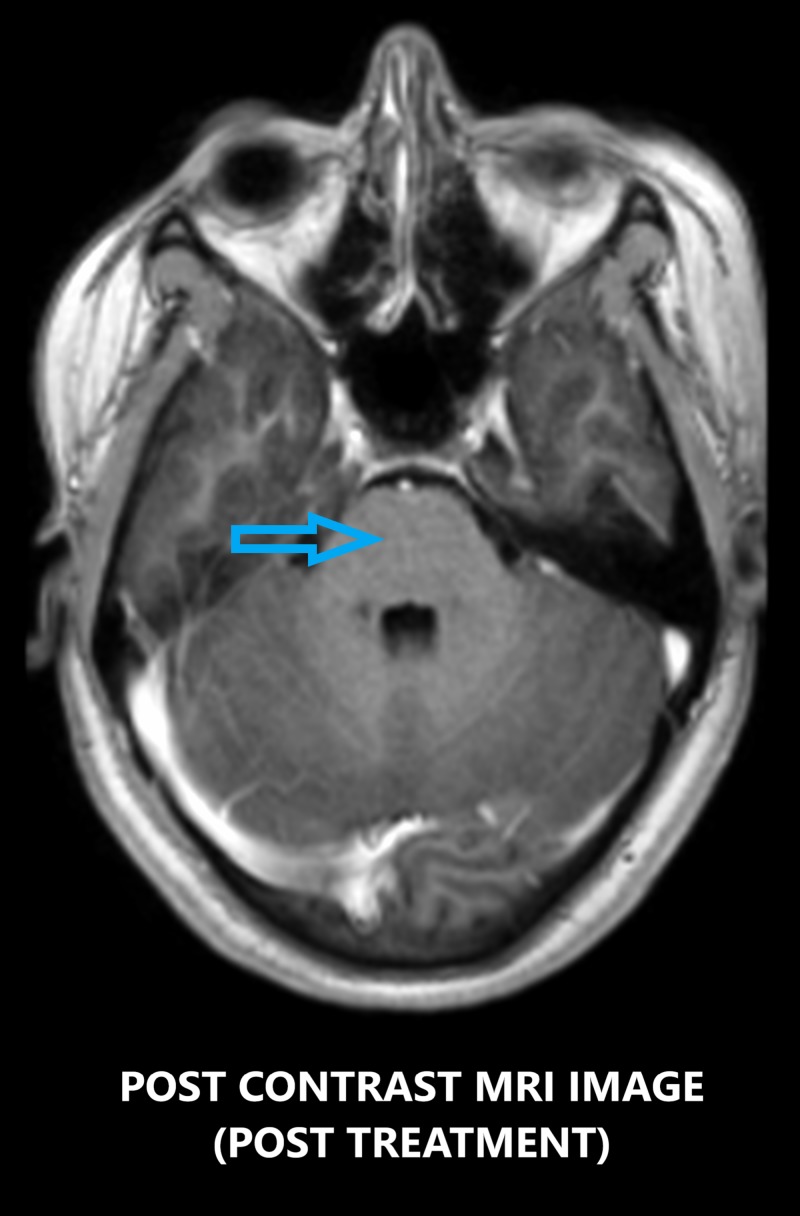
Contrast-enhanced axial MRI image showing the region of the pons after treatment with high-dose steroids depicting no punctate lesions in the brainstem (blue arrow). MRI: magnetic resonance imaging
